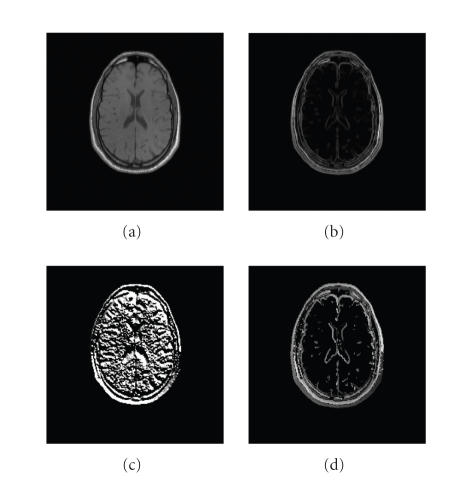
A slice of 3D GCM of MRI T1. (a) Original image, (b)
